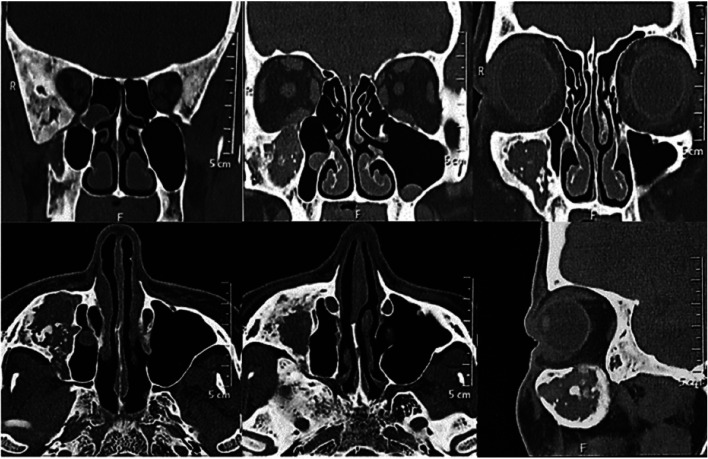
Computed tomography (CT) performed 6 years ago showed an extensive and expansive lesion with a mixed aspect such as “ground glass”. The lesion involved the maxilla, greater sphenoid wing, temporal, frontal, pterygoid process, floor and lateral wall of the orbit of the right side. It was also observed an extensive hypodense and unilocular area in the right maxilla, which caused a remodeling of the lateral and inferior walls of the ipsilateral maxillary sinus, decreasing its dimensions. Moreover, a cortical thickening of orbital floor and anterior wall of the maxillary sinus was observed. Similar lesions (in smaller size) were also observed in the sphenoid bone.
